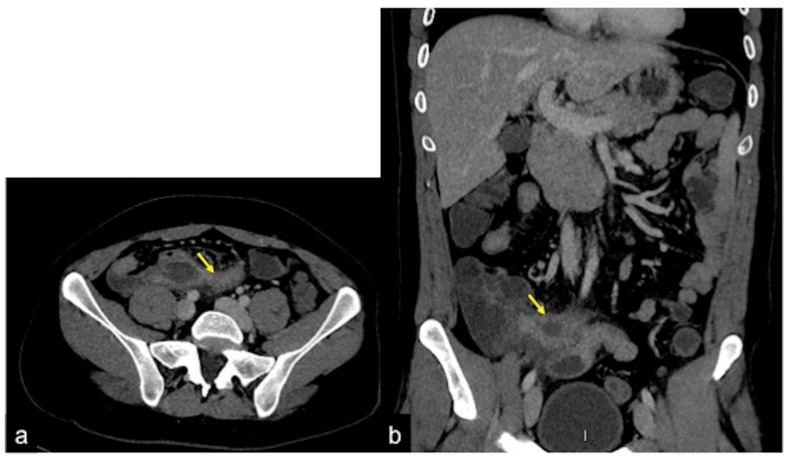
CTA axial (a) and coronal (b) images in the venous phase show inflammatory stenosis of terminal ileum ((a) arrow) in Crohn’s disease complicated by an abscess ((b) arrow).
